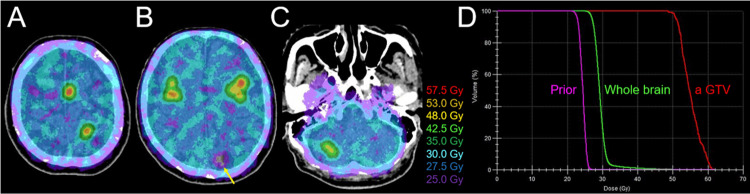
Dose distributions and dose-volume histograms for VMA-based WBRT with simultaneously integrated boosts to 17 lesions The images show dose distributions (A, B, C); and DVHs (D) (A-C) The representative isofill doses are superimposed onto non-CE CT images. The dose in the previously irradiated region was intentionally moderately reduced to control potential dissemination and to reduce the risk of radiation injury (arrow in B). The doses to the bilateral auditory apparatuses are significantly reduced, compared with those for conventional opposed-field WBRT. (D) The DVHs of the largest GTV (0.33 cm^3^) in the left frontal lobe, the whole brain, and the prior irradiated region (Prior) are representatively shown. The D_98%_ and D_50%_ of the GTV are 50.5 Gy and 54.9 Gy, where sufficient GTV coverage with 53 Gy (BED_10_ >80 Gy) is intentionally withheld to reduce the risk of radiation injury. The D_98%_ and D_50%_ of the whole brain are 26.6 Gy and 29.2 Gy, and 30 Gy encompasses 28% of the whole brain. The D_98%_, D_50%_, and D_2%_ of the Prior region are 22.4 Gy, 24.5 Gy, and 26.1 Gy, respectively GTV: gross tumor volume; DVHs: dose-volume histograms; CE: contrast-enhanced; WIs: weighted images; VMA: volumetric-modulated arc; WBRT: whole-brain radiotherapy; BED_10_: a biologically effective dose based on the linear-quadratic formula with an alpha/beta ratio of 10; D_X%_: a minimum dose encompassing at least X% of a target volume
